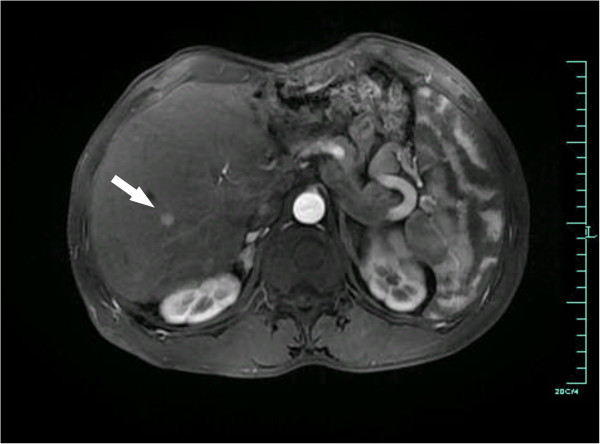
Artery phase of enhanced abdominal MRI 2 years after living donor liver transplantation. The white arrow indicates a 0.7-cm nodule of hepatocellular carcinoma (HCC) with arterial enhancement in segment VI of the allograft.
